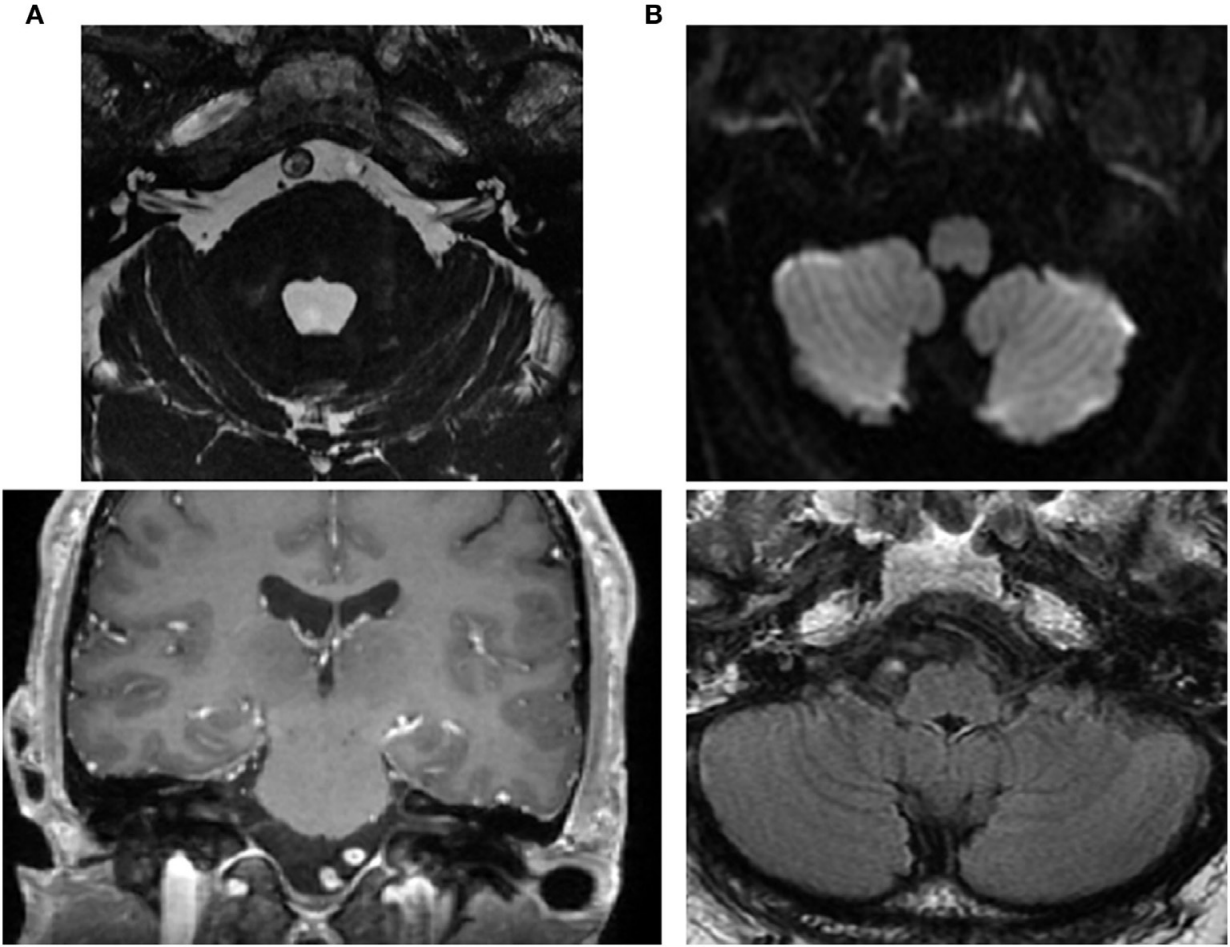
Brain MRI. (A) Normal internal auditory canals as visualized *via* axial FIESTA (upper) and coronal T1 with contrast (lower). (B) Normal inferior and medial vestibular nuclei at the level of the medulla (axial diffusion—upper) and FLAIR (lower).
